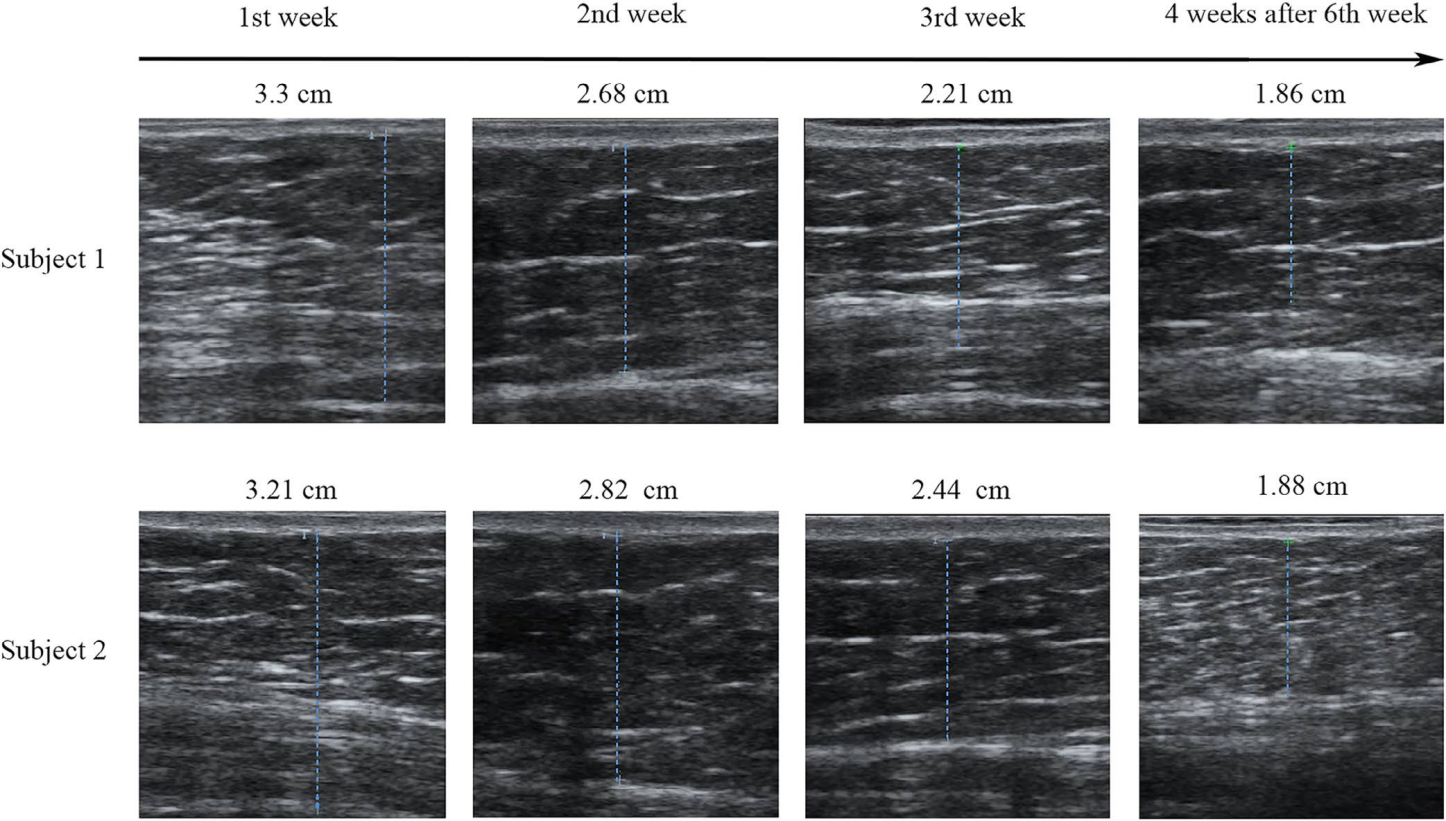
Ultrasonographic evaluation of subcutaneous adipose tissue thickness over time in two subjects. The figure shows ultrasonographic images of abdominal subcutaneous adipose tissue thickness at four time points (from left to right): 1st week, 2nd week, 3rd week, and 4 weeks after the 6th week. For Subject 1 (upper row), the thicknesses are 3.3 cm, 2.68 cm, 2.21 cm, and 1.86 cm, respectively. For Subject 2 (lower row), the thicknesses are 3.21 cm, 2.82 cm, 2.44 cm, and 1.88 cm, respectively.
